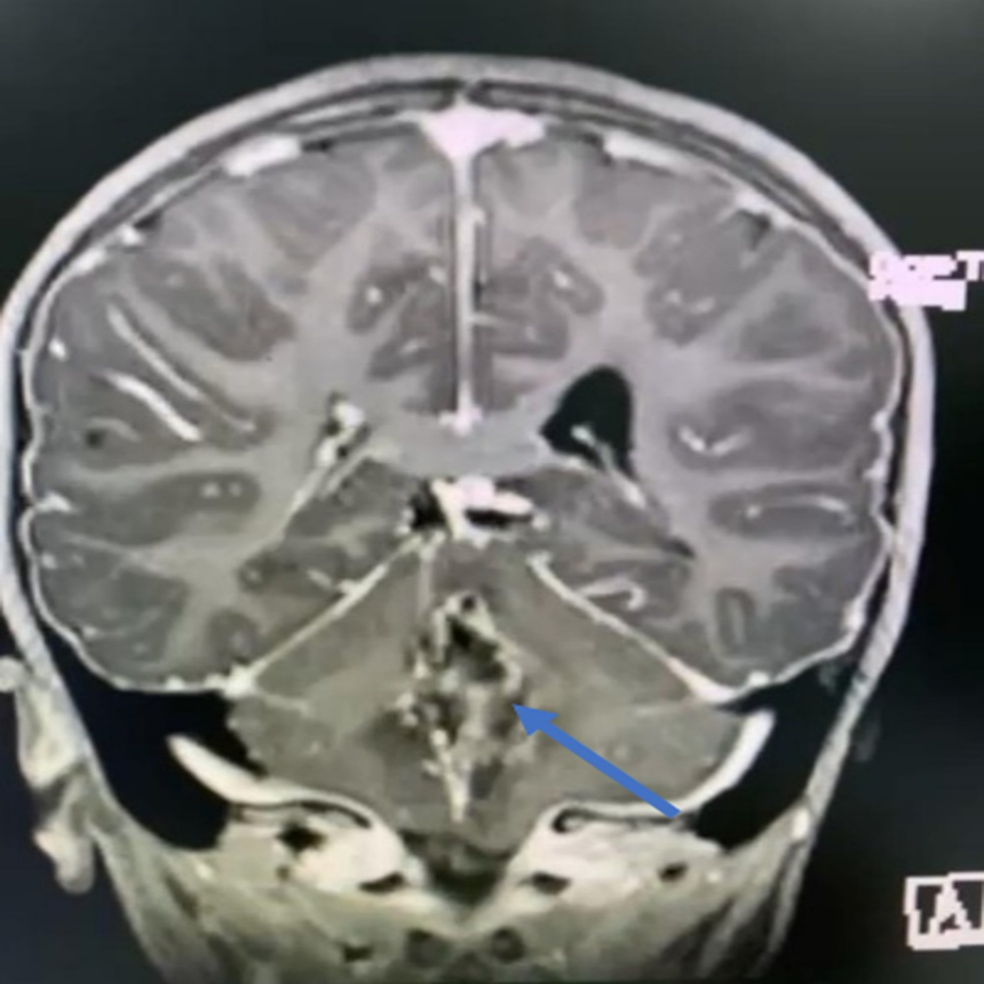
Postoperative image - MRI brain coronal cut
